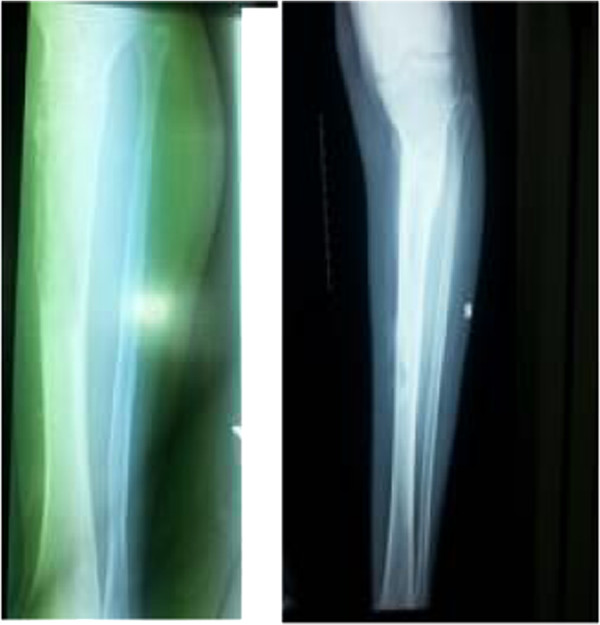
Control x-rays of the right leg taken at four months (left) and two years (right) after definitive management.
